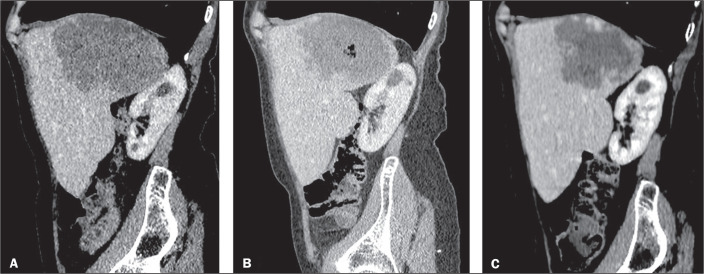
Contrast-enhanced abdominal computed tomography, with sagittal
